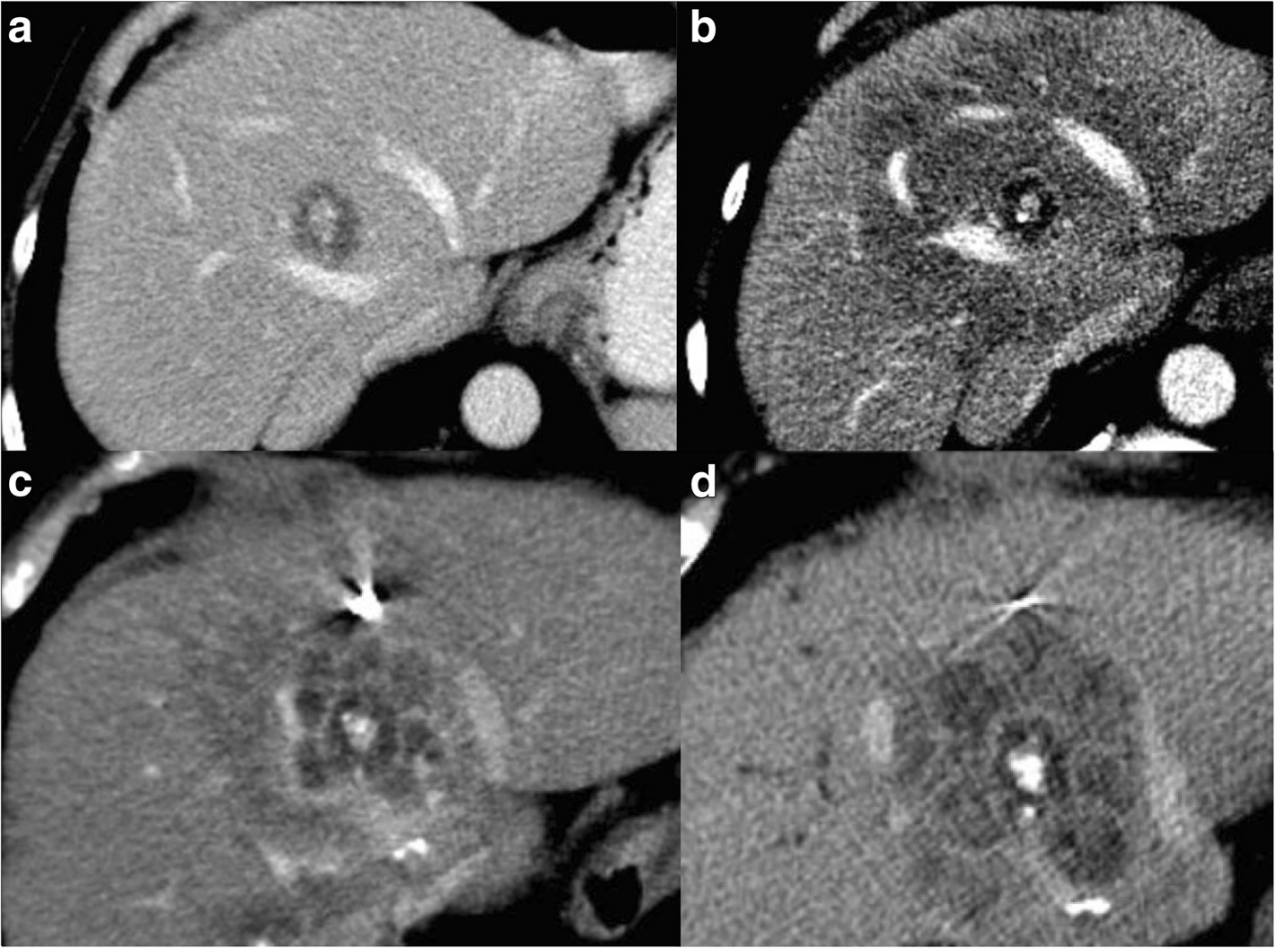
Progressive lesion after SBRT; a liver metastasis with a calcified center before treatment (a), 2 months later (b) we observe the changes of the initial phase as described, 4 months (c) and 6 months later (d) CT slices show the Daisy Sign: a lobulated thick peripheral enhancement.
